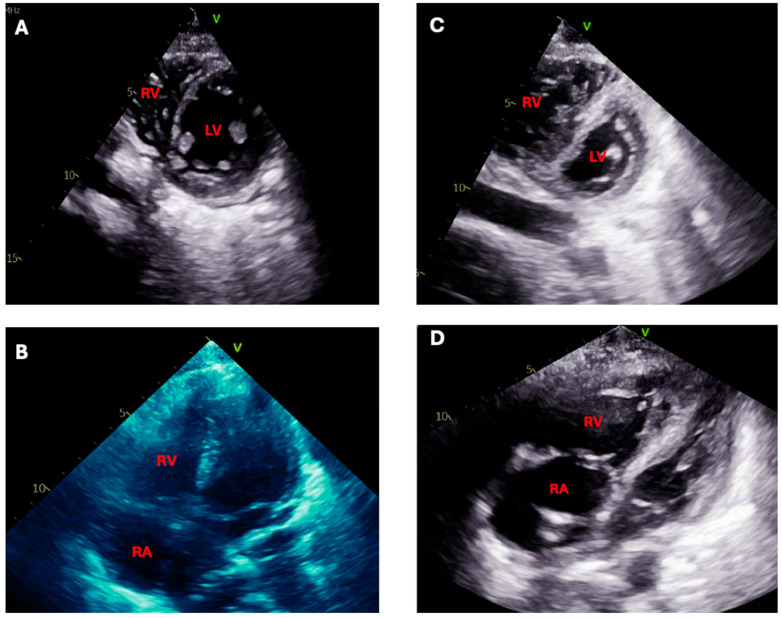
Echocardiographic images from a patient with rapidly progressive interstitial lung disease admitted to the ICU. Images (A,B), taken at initial presentation, show a normal right ventricle (RV), left ventricle (LV), and right atrium (RA). Five days later, repeat imaging reveals acute right ventricular dilation in both the parasternal short-axis (image (C)) and apical 4-chamber (image (D)) views, consistent with rapid development of right ventricular failure in the setting of ILD exacerbation.
